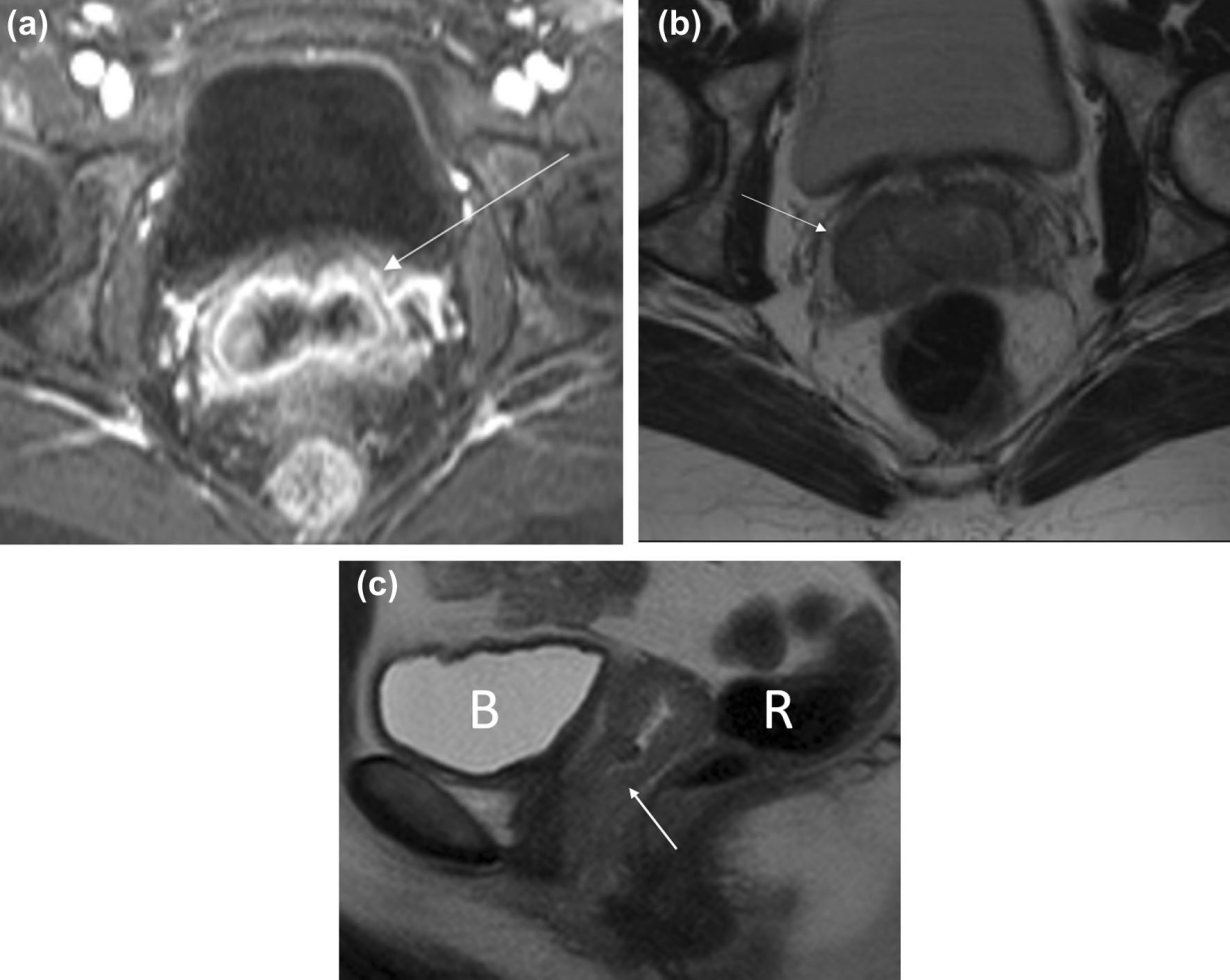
51-year-old woman with a history of cervical cancer who developed FIGO stage 1 squamous cell carcinoma of the vagina. a Axial T1-weighted fat-saturated contrast-enhanced MR image shows thickening of the vagina (arrow). b Axial T2-weighted MR image shows no paravaginal involvement (arrow) with an intact vagina. c Sagittal T2-weighted images show that the tumor is confined to the vaginal wall with no involvement of the rectum (R) or bladder (B)
